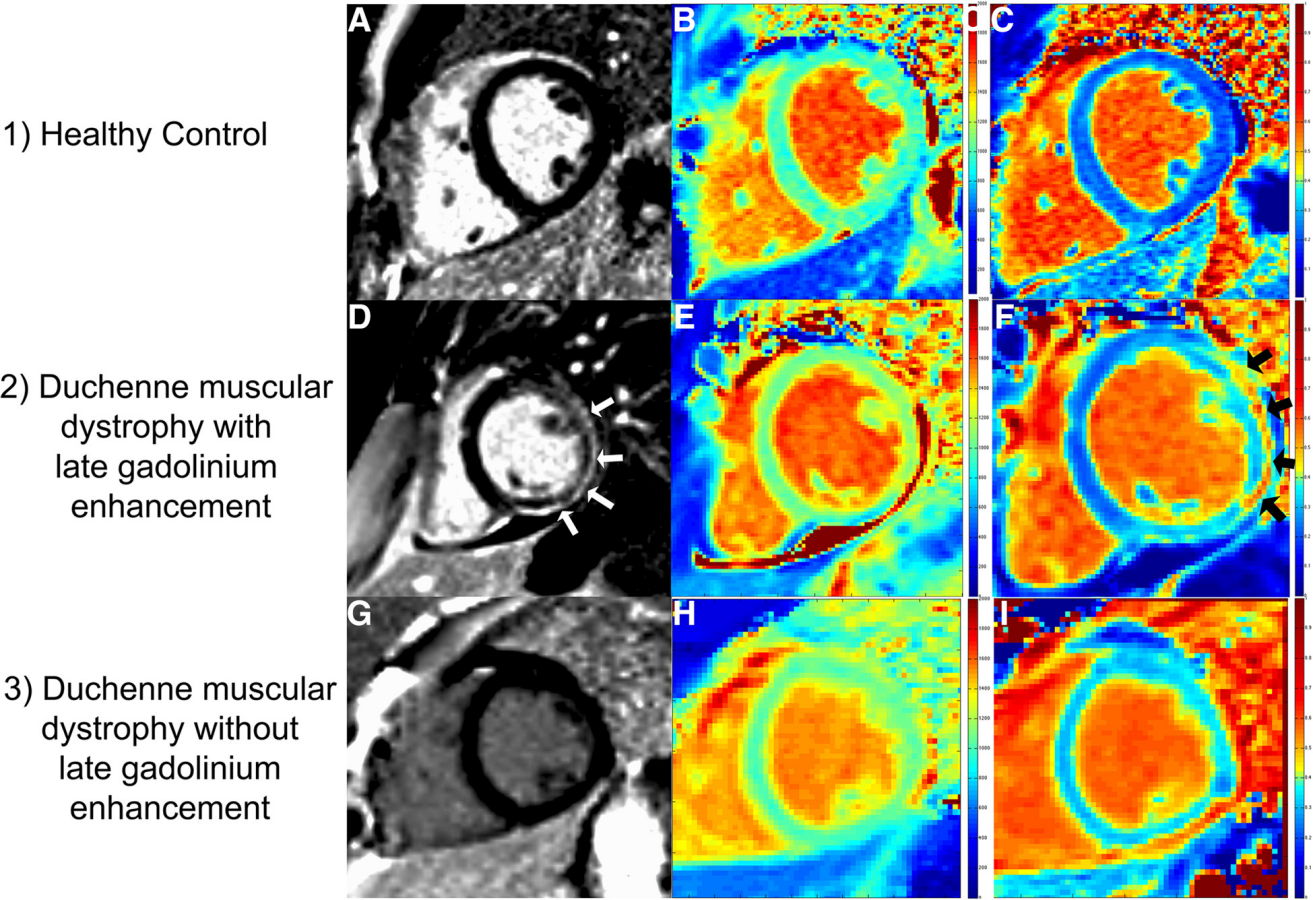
Example cardiac MRI images. 1) Healthy control without late gadolinium enhancement (LGE) (a), normal native T1 (b), and ECV (c) values. 2) Duchenne muscular dystrophy (DMD) participant with significant LGE (d) (arrows); native T1 map (e) demonstrates increased T1; ECV map (f) demonstrates similar areas of elevated ECV (arrows) as the LGE. 3) LGE image in DMD participant without LGE (g) but with diffusely elevated native T1 (h) and ECV (i) suggesting extracellular matrix expansion
